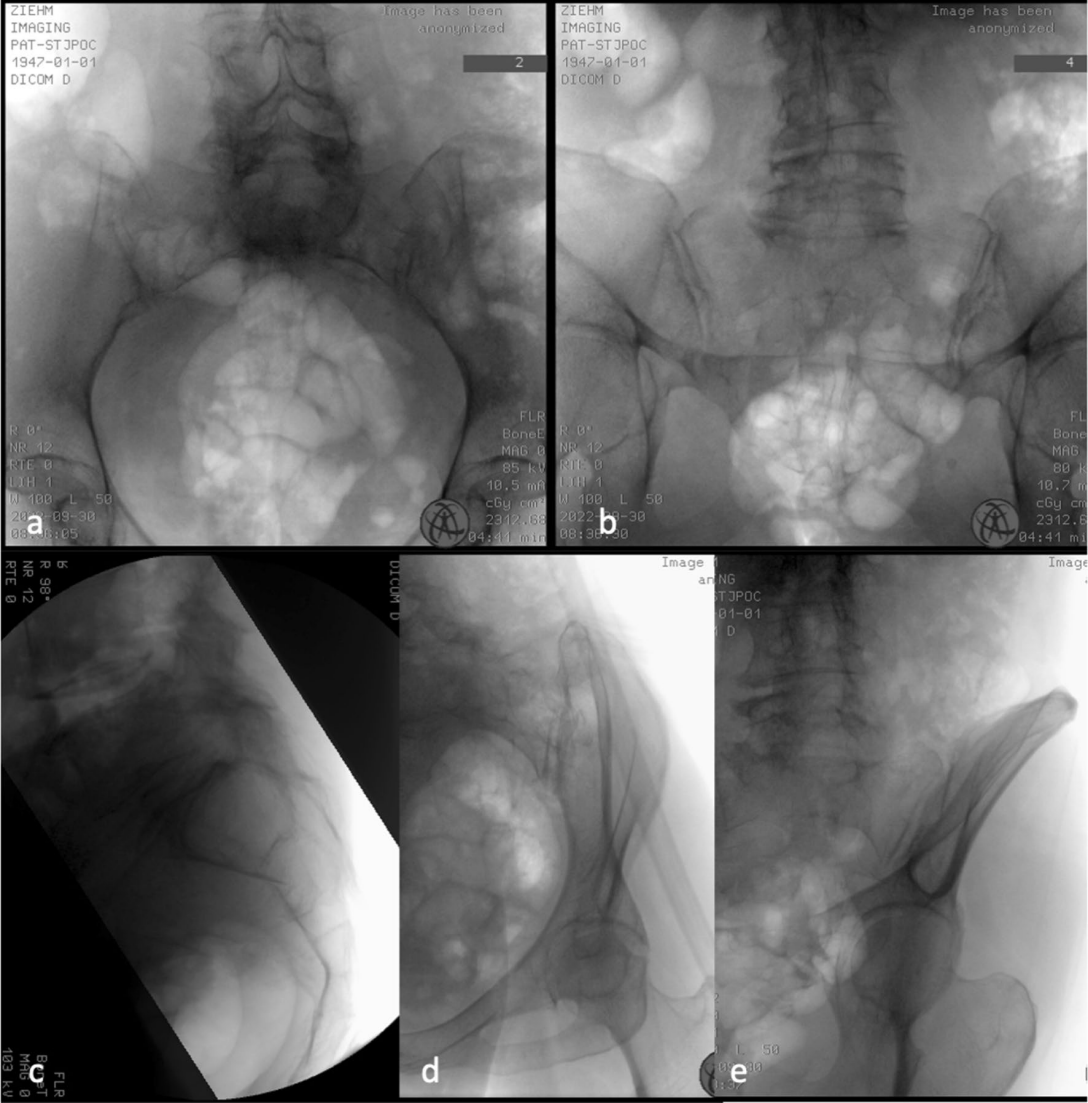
Relevant projections for intraoperative planar radiography: inlet (a), outlet (b), strict lateral (c); oblique view of obturator + inlet (d), oblique view of obturator + outlet (e)
